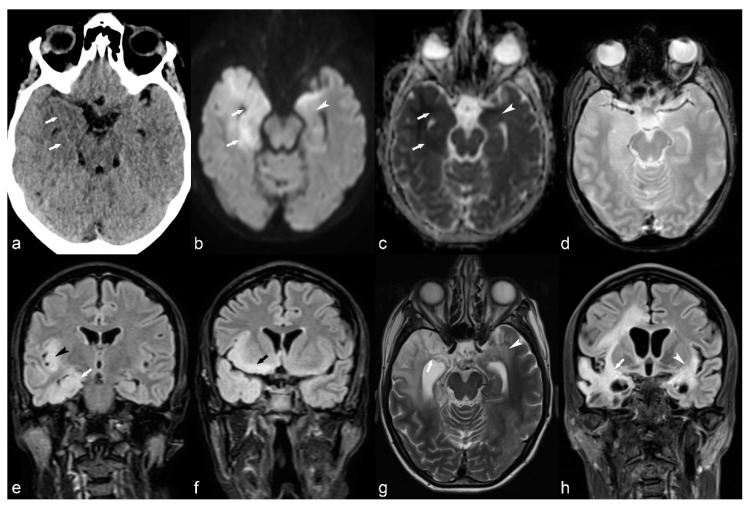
35-year-old woman with herpes encephalitis. Non-contrast CT (a) shows low attenuation in the right mesial temporal region (white arrows). DWI b1000 (b) and ADC map (c) confirm bilateral but asymmetrical restricted diffusion in the mesial temporal areas (white arrows and arrowhead). No haemorrhage is identified on T2* (d). There are signal abnormalities on FLAIR (e,f), most pronounced in the right mesial temporal lobe (white arrow), right insula (black arrowhead) and right frontal lobe (black arrow). MRI performed 2 months later (g,h) shows bilateral areas of encephalomalacia (white arrows and arrowhead), more pronounced on the right, despite treatment.
